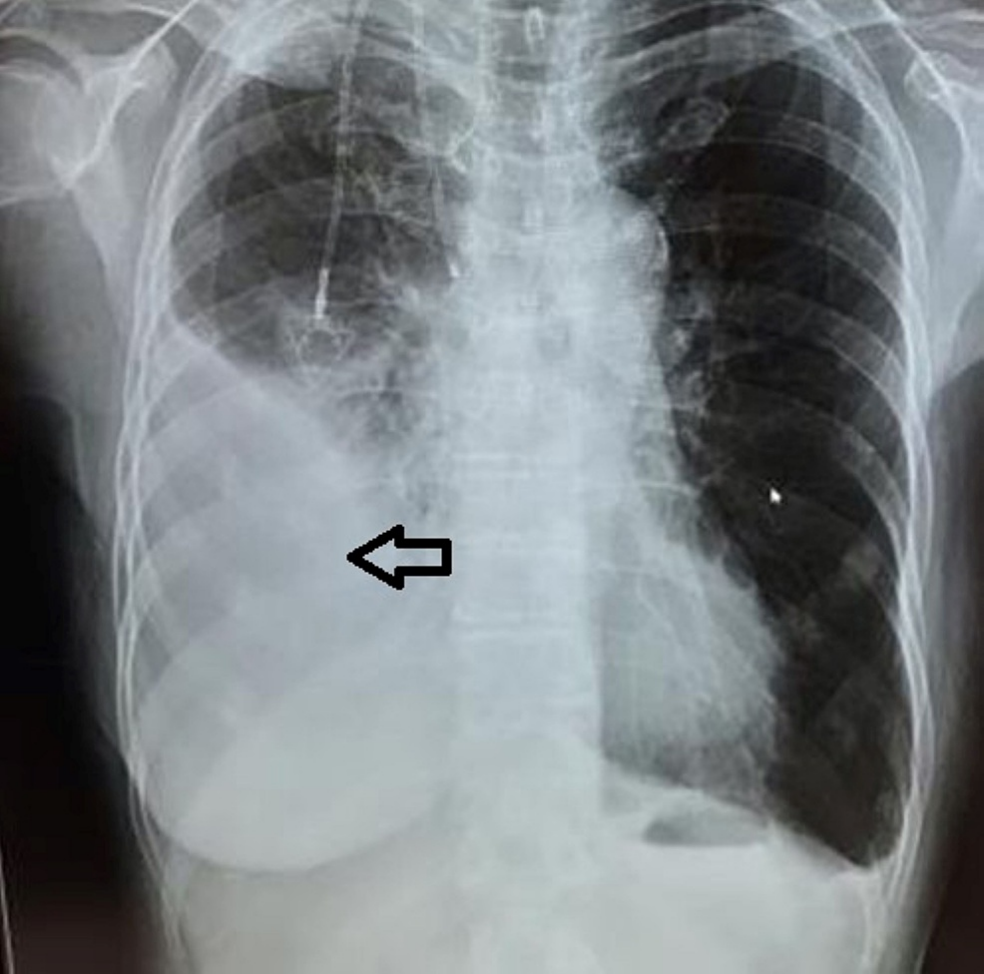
CXR showing pleural effusion due to breast cancer dissemination CXR: chest X-ray
